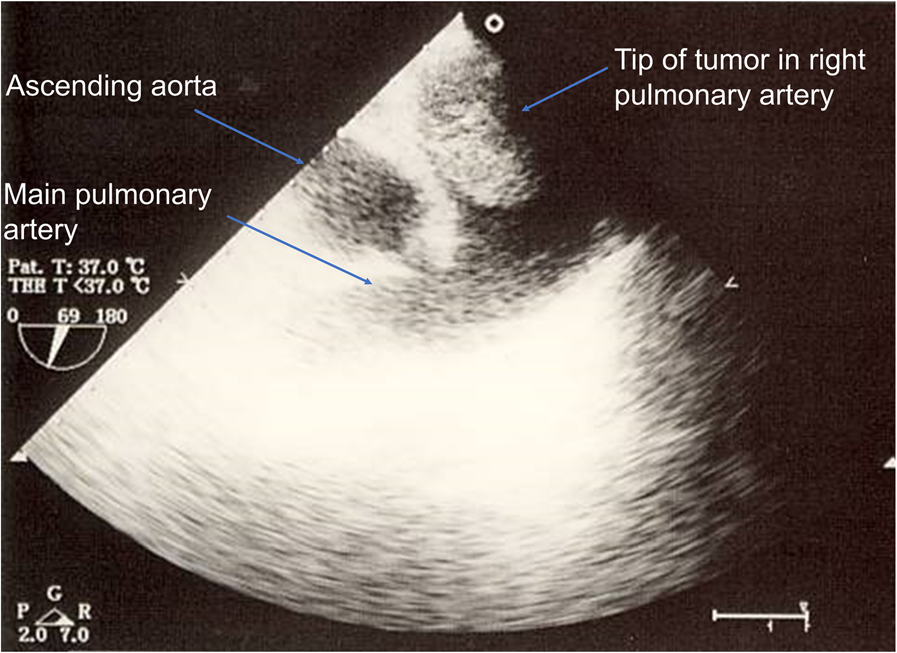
The tumor in the pulmonary artery was confirmed on intraoperative transesophageal echocardiography. The transesophageal echocardiography revealed a mobile mass, extending from the left main pulmonary artery (PA) to the right PA, which obstructed the lumen of the right PA (as shown on the mid-esophageal aortic valve short-axis view)
